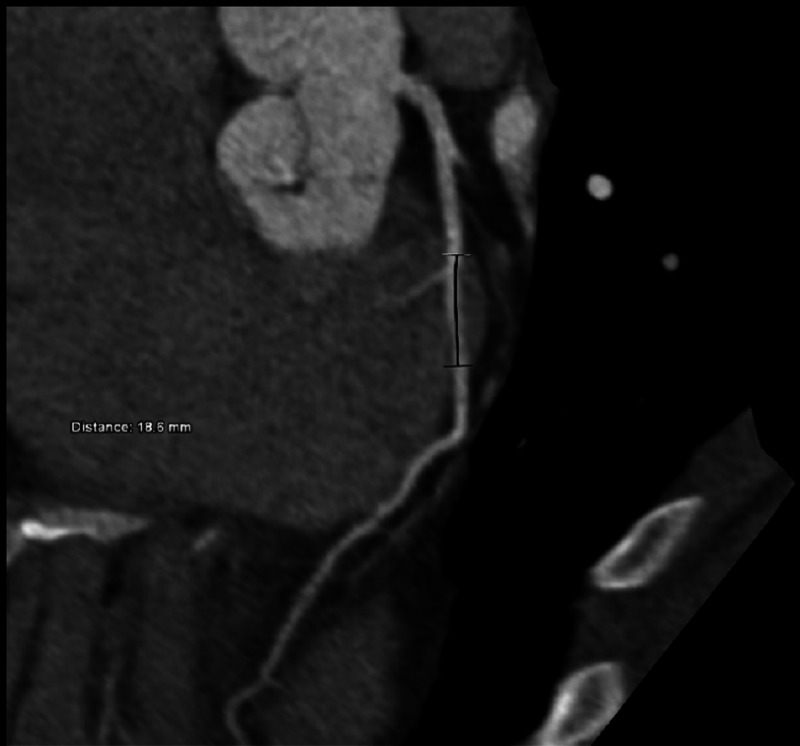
CTA of the patient The image showed mid-LAD deep myocardial bridging, the tunneled segment of about 1.8 cm with about 5 mm depth in the myocardium CTA: computed tomography angiography; LAD: left anterior descending
